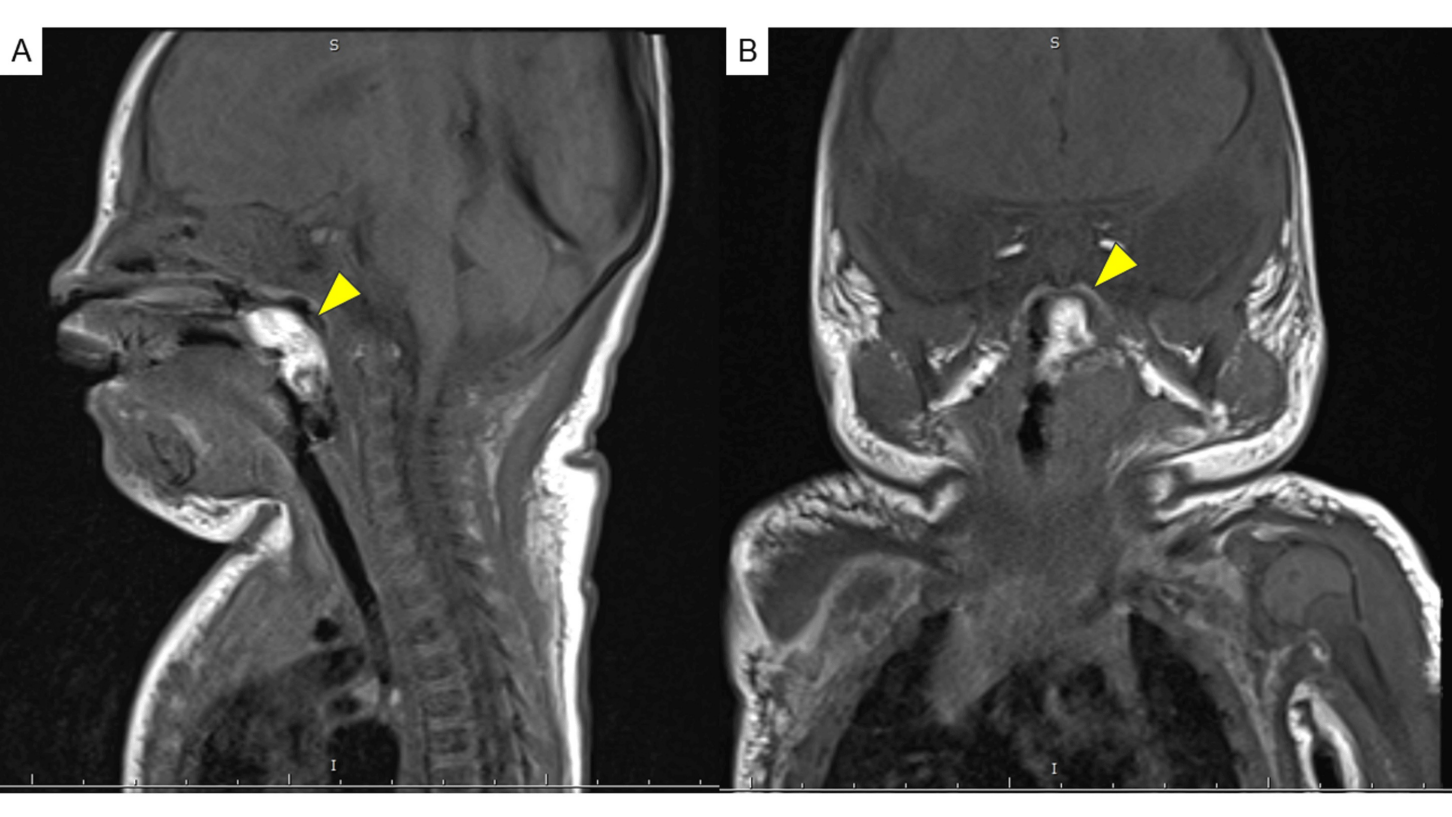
Magnetic resonance images of the neck The images reveal a mass (arrow) near the left uvula, surrounded by fatty components that showed high signal intensity on T1-weighted imaging, the central area of which is equivalent to that of a muscle with a pedicle (A, sagittal view; B, coronal view).
